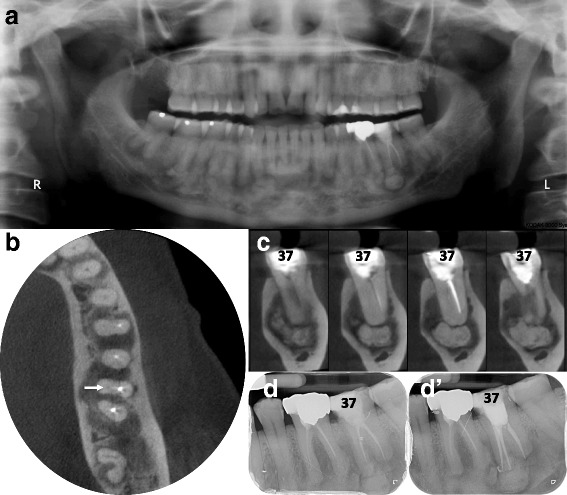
Stage 2 FOD with concomitant endodontic periapical lesion on symptomatic tooth 37. Diffuse mixed (radiolucent and radiopaque) periapical lesions are depicted on panoramic radiography (a). High-resolution/small field of view cone-beam CT allows axial (b) and bucco-lingual cross-sections (c) highlighting the lack of endodontic treatments and unobturated mesio-lingual canal (*white arrows*) associated with voluminous peri-radicular mixed-density images. (d, d’) Following endodontic retreatment tooth 37 became asymptomatic but the 6 months post-operative intra-oral x-ray (d’) was unable to show usual signs of alveolar bone healing as compared to the preoperative x-ray (d) because of superimposed FOD lesions
